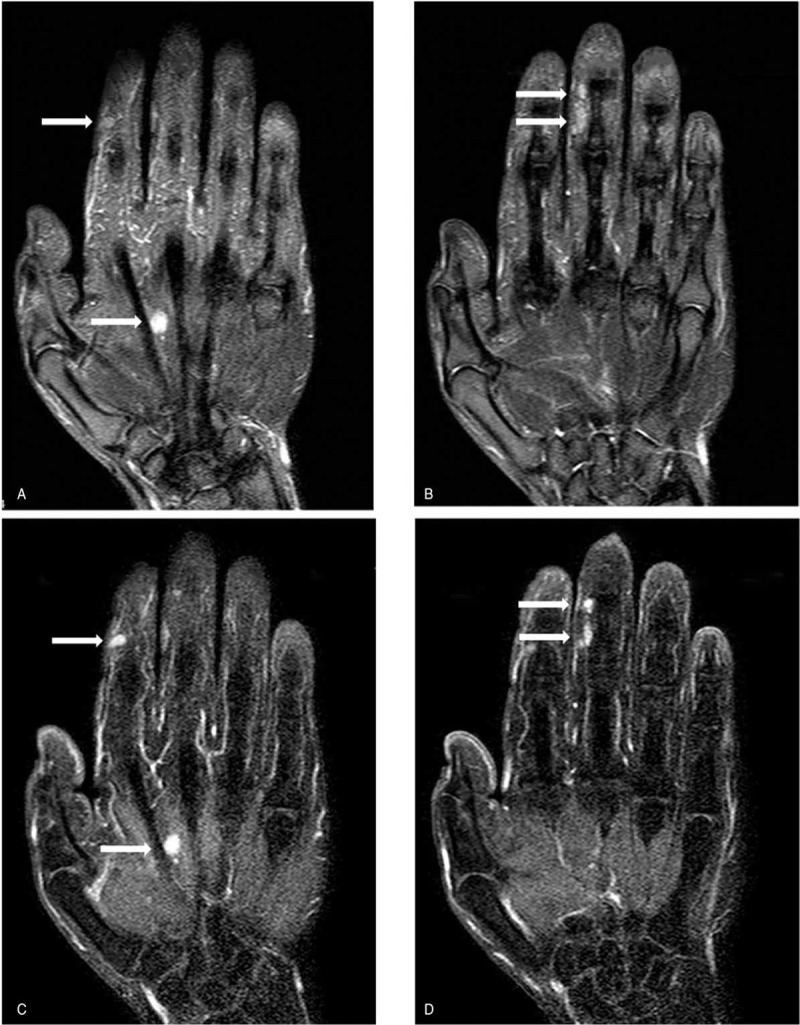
Coronal MR images showing round lesions (A-B): T2-weighted multi echo data image combination sequence (TR/TE: 2500/60) demonstrated four nodules of high intensity signal (arrows). (C-D): The tumors showed significant enhancement after administration of contrast agent (arrows).
